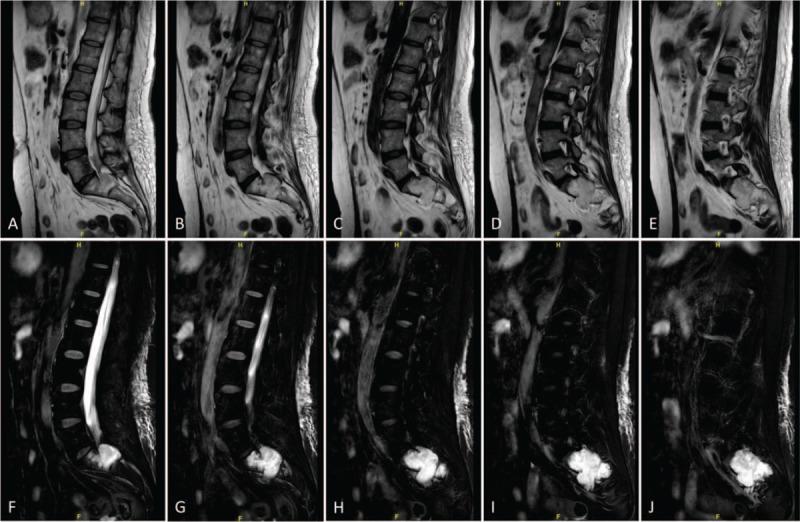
(A–J) Preoperative sagittal MRI scan revealing the density of soft tissue with obvious bony destruction in the sacrum, and significant spinal cord compression secondary to the mass, with increased marrow infiltration of the sacrum.
